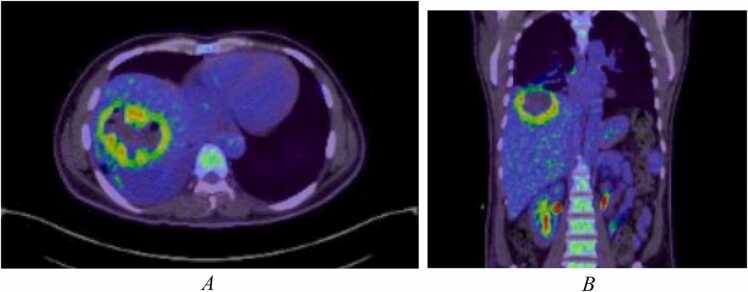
A and B PET CT FDG uptake scan demonstrates a large lesion in the right liver lobe segment VII, VIII measuring 7 cm by 7.5 cm with increased FDG uptake along its peripheral thick wall with air locules seen within the lesion.
